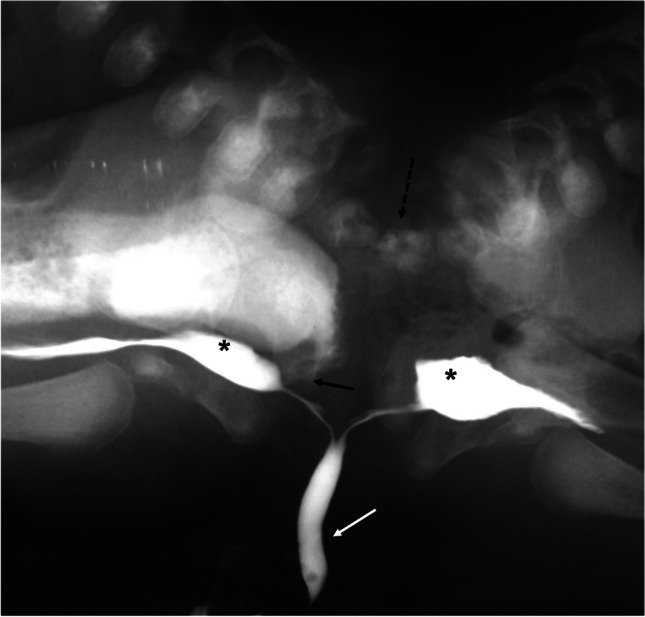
An anteroposterior pelvic radiograph obtained during micturating cystourethrography in 4-week-old pygopagus conjoined twin boys shows a single urethral channel (*white arrow*) with two separate urinary bladders (*asterisk*s). Note the vertebral fusion (*broken arrow*) and rectourethral fistula in the right twin (*black arrow*)
